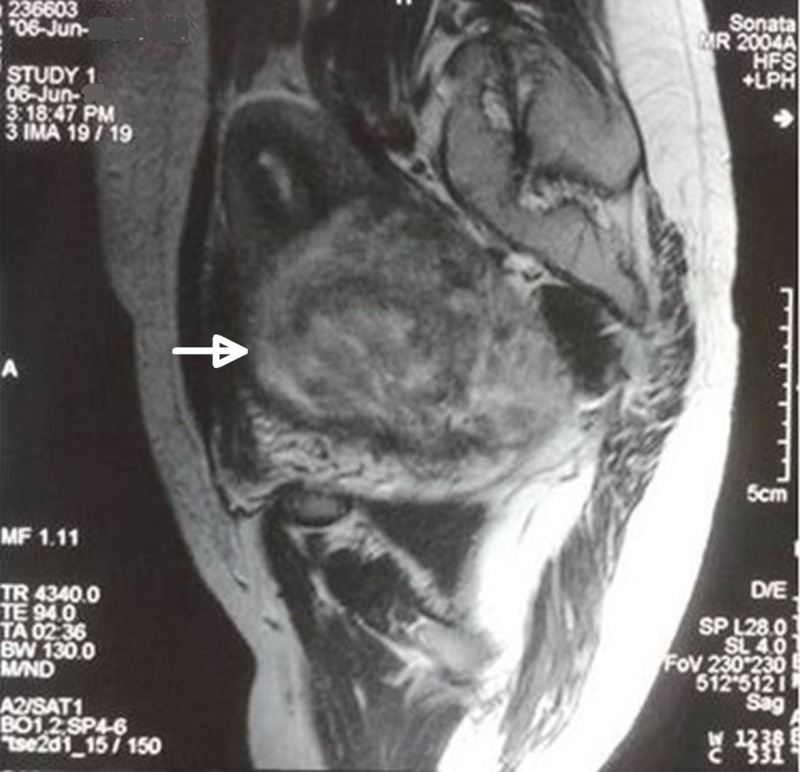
MRI image (Case 3) T2 weighted MRI image (sagittal view) showing a huge cervical fibroid with whorled appearance displacing the uterus anteriorly and upwards (white arrow)
